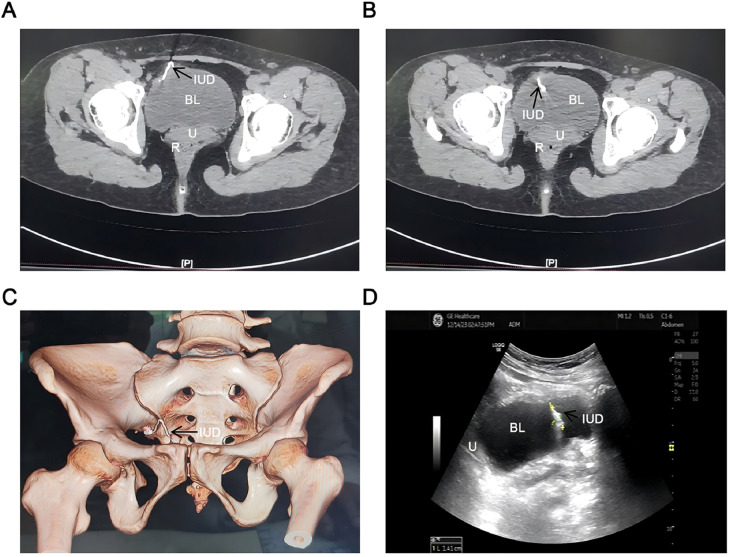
Assessment of the relationship between the IUD and the bladder before operation. (A) and (B) The relationship between the IUD and the bladder, uterus, and rectum was assessed using CT imaging; (C) The position relationship between the IUD and pelvis was evaluated by three-dimensional stereoscopic imaging; (D) The position relationship between the IUD and bladder was evaluated by color ultrasound. BL, bladder; U, uterus; R, rectum; IUD, intrauterine device.
